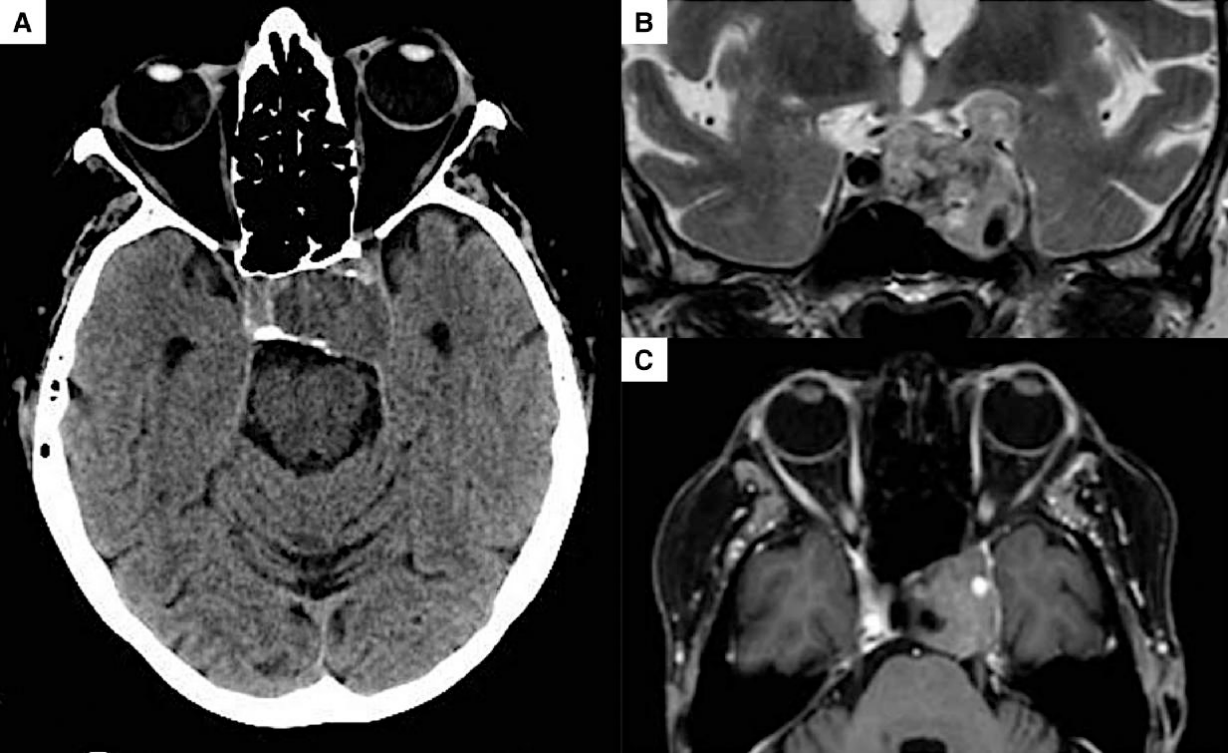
A, Axial CT image of sellar-parasellar mass, without contrast medium. B, and C, Coronal T2 weighted image and axial T1 postcontrast image of sellar-parasellar mass, with left cavernous sinus invasion and extension to temporal lobe.
